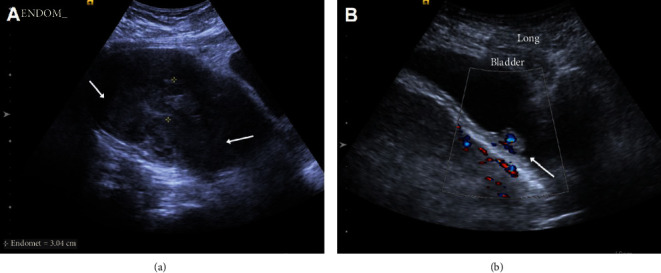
Grayscale ultrasound image of the uterus (a) demonstrates a heterogeneous uterine echotexture with poorly defined masses (arrow, (a)). The endometrial stripe is thickened (clippers, (a)) and measures up to 3.0 cm. Color Doppler image (b) shows a soft tissue mass within the posterior wall of the urinary bladder (arrow, (b)) with vascularity.
